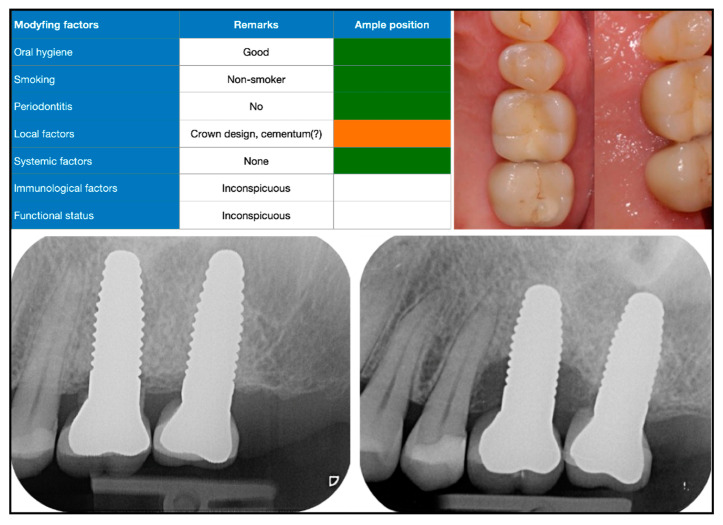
Initial findings in healthy patient with two implants in maxilla (risk profile or traffic light, top left). After healing, two crowns were cemented intraorally (initial radiograph, bottom left). Despite clinically and visually inconspicuous status, mesial implant showed rapidly progressive bone resorption a short time later (X-ray, bottom right).
